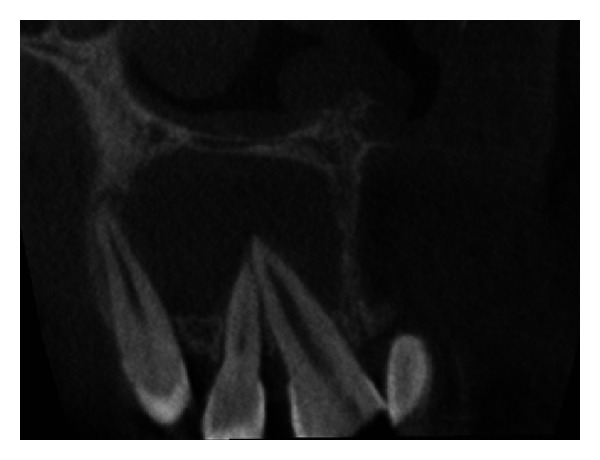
Pre-operative CBCT image in coronal plane.
